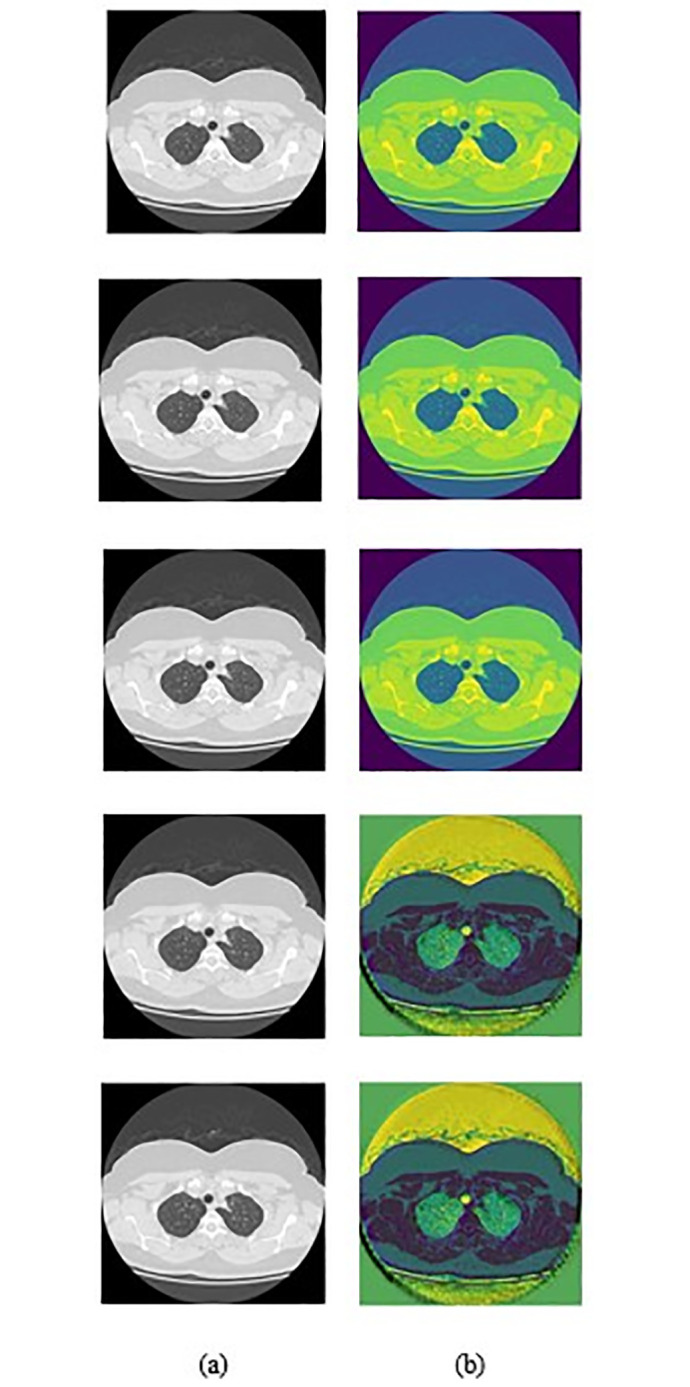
Image Results for Lung Disease Segmentation and Severity Classification using CT Images a) Input Images and b) Improved Weiner Filtering.
